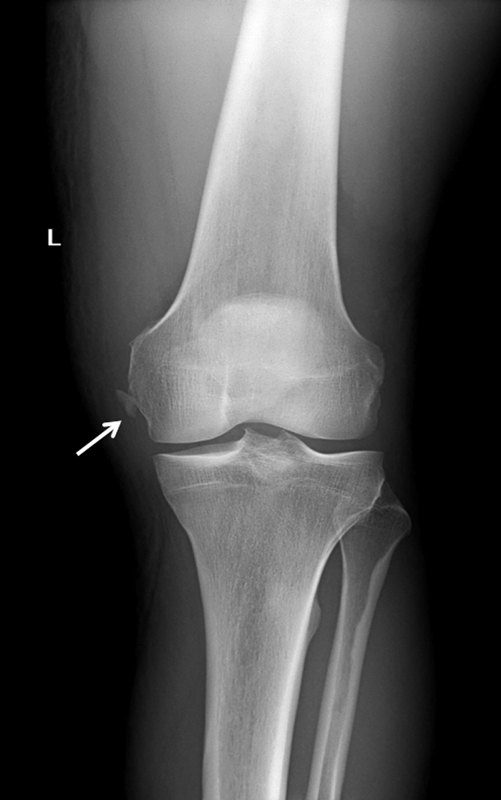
Anteroposterior X-ray of the right knee showing an avulsion fracture on the medial femoral condyle (arrow).
